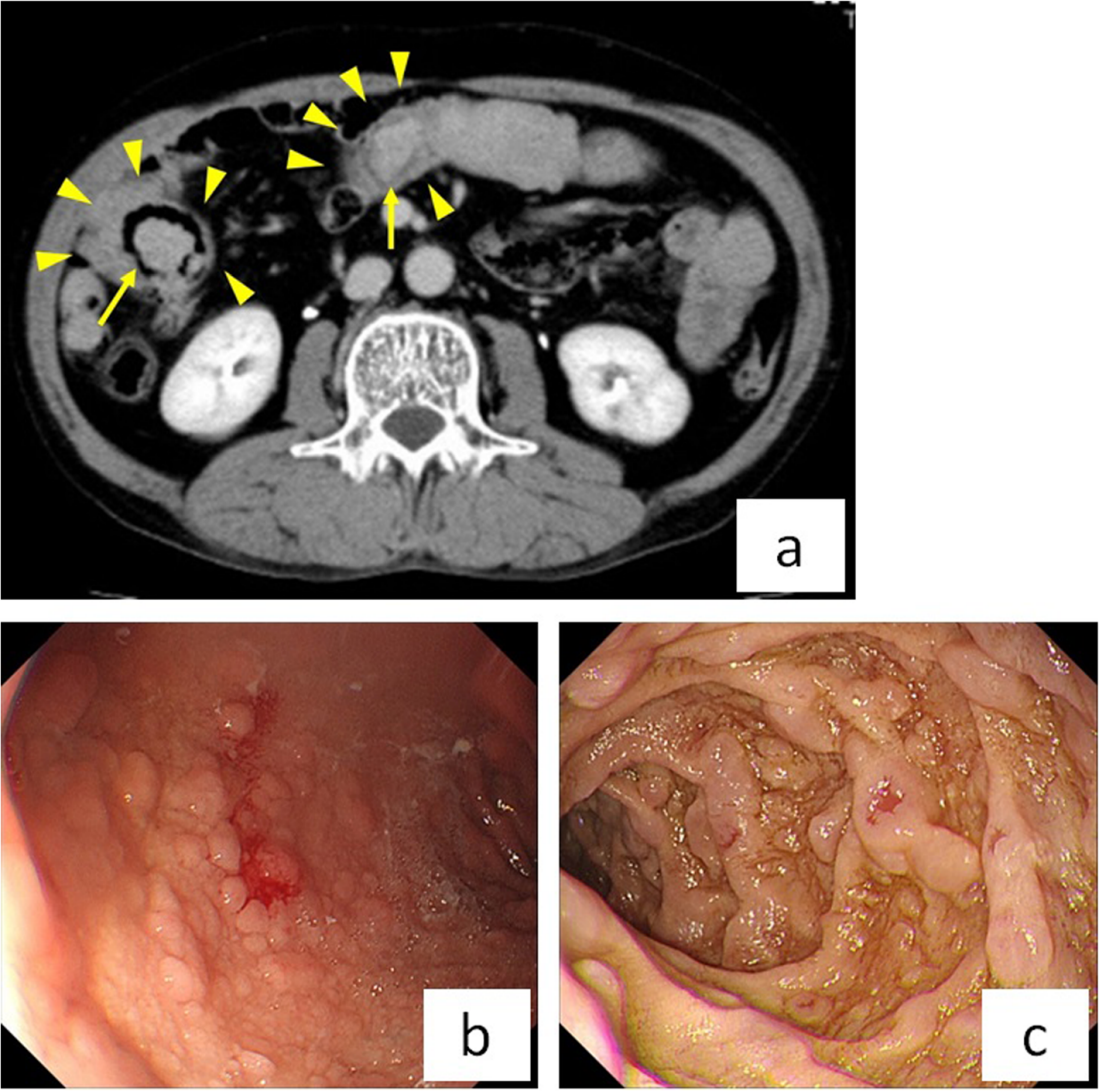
a In the small intestine, CT shows multiple intussusceptions (arrowheads) and polyps (arrows). b, c Gastrointestinal fiberscopy shows multiple polyposis in the stomach b and duodenum c
